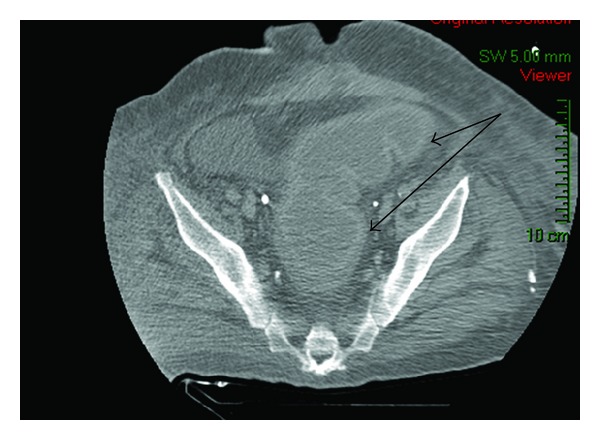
CT abdomen of a patient with Clostridium difficile colitis. The image shows dilated descending loop with thickening of wall (see black arrows).
